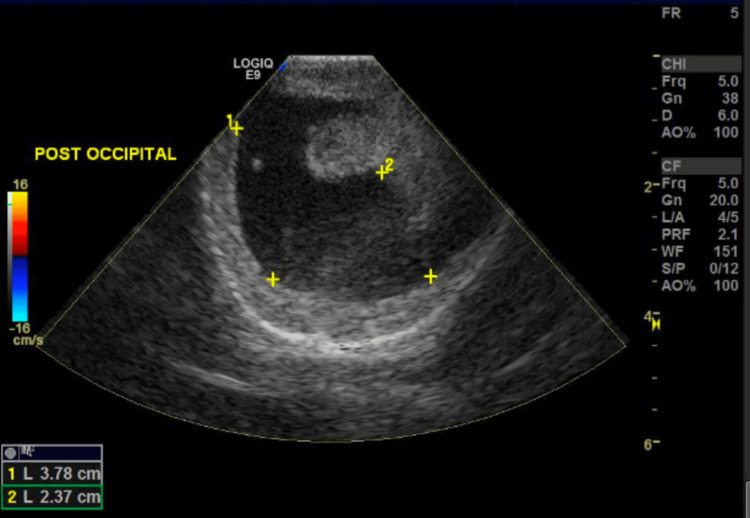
Transcranial grayscale ultrasound image of an occipital lesion consistent with an encephalocele
